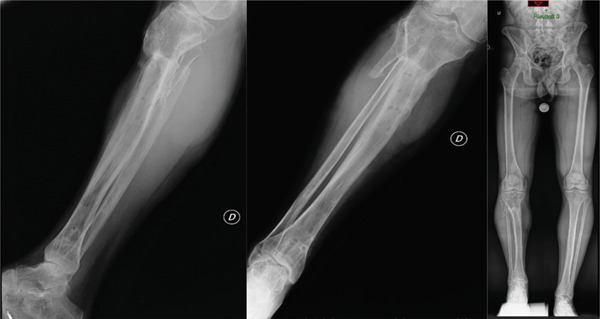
Patient′s condition after removal of FE, full‐length standing radiograph of the lower limbs with a 6‐cm lift on the right side.
